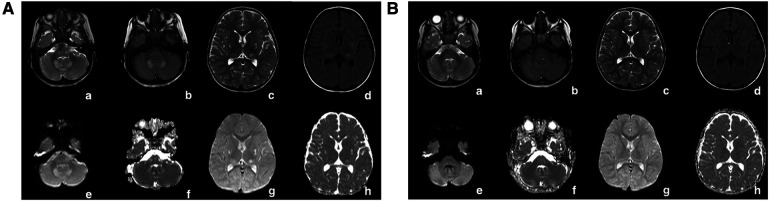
(A) In patient 1, at the onset of the disease (1 year and 8 months old), the cerebral MRI showed symmetrical patchy long T2 signals in the cerebellar dentate nucleus, bilateral basal ganglia, and thalamus. (B) In patient 1, the signal of long T2 in the brain improved gradually after vitamin B1 treatment for 1 year. The long patchy T2 signals were significantly attenuated on T2WI (a,e), T2-FLAIR (b,c), and DWI (c,g) sequences.
